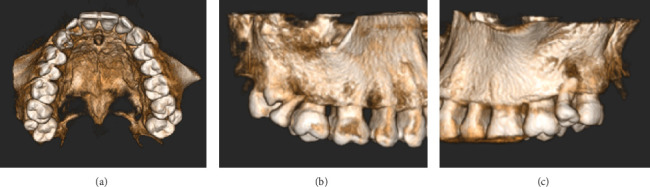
(a) Occlusal view of the maxillary dentition from a three-dimensional reconstruction of the CBCT scan. (b, c) Sagittal views of the (b) right and (c) left maxillary hemiarches.
